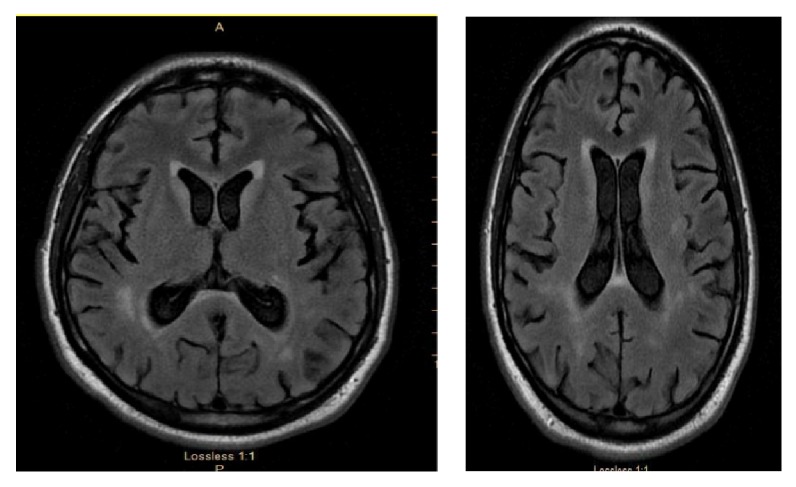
Initial MRI showed diffuse, patchy foci of increased FLAIR signal in the periventricular, deep, and subcortical white matter.
